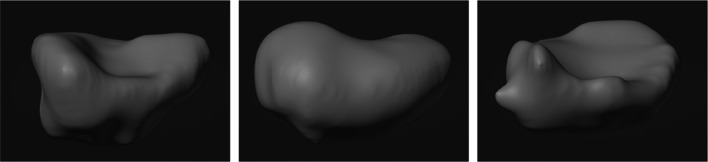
Anatomically accurate 3D bladder models with different filling states obtained by CT scans
